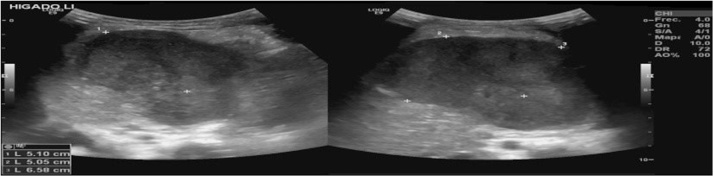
Two ultrasound images of the same tumor. Rounded hypoechoic mass between the left lobe of the liver and the spleen. Approximately 52 × 59 × 56 mm.
